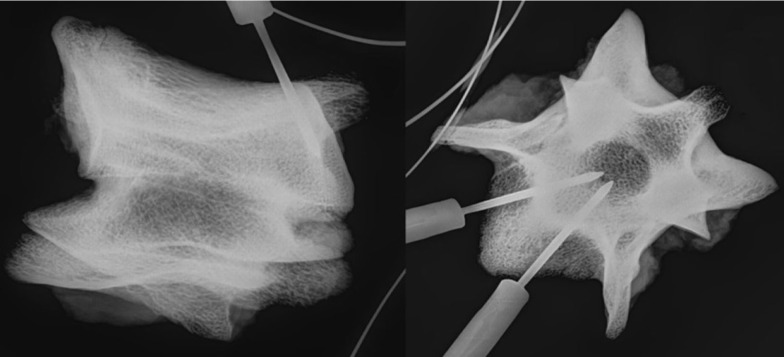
Radiograph showing the location of the temperature probes within the vertebra in a lateral (left) and axial (right) view.
